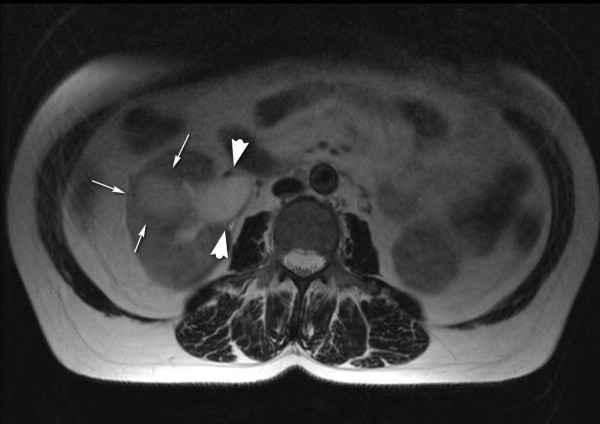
T2-weighted axial magnetic resonance imaging scan showing a high-signal (fluid) cortical mass (arrows) with an irregular low-signal capsule. The collecting system is dilated (arrowheads).
